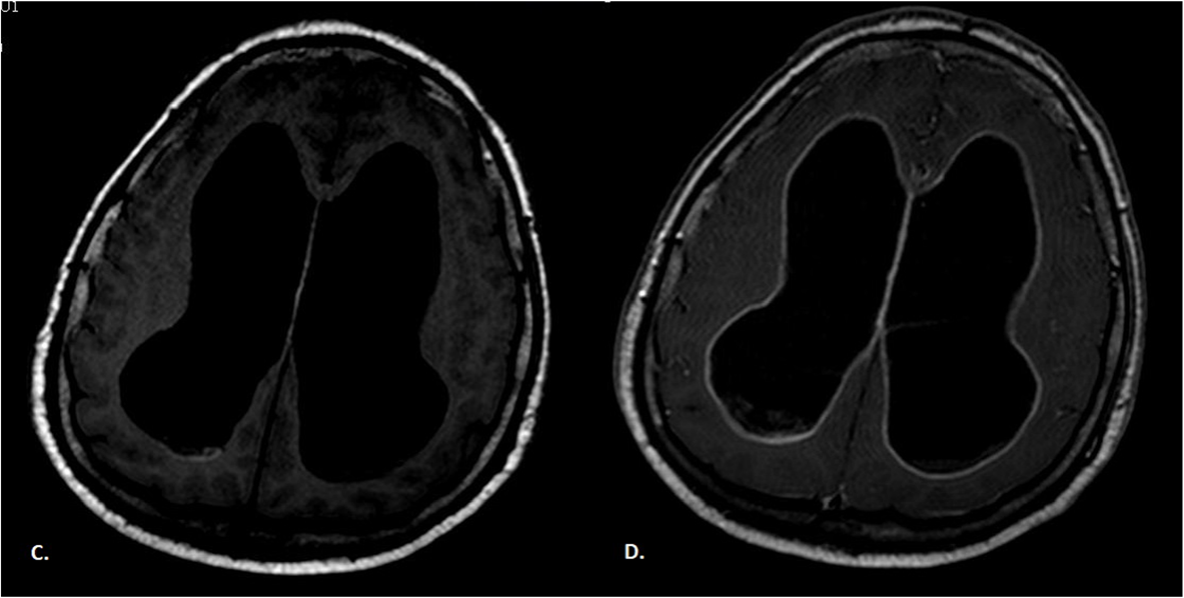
Brain MRI T1-weighted imaging without (c) and with (d) gadolinium contrast enhancement. Hydrocephalus as a consequence of disease progression. Hyper-intensity on T1 was due to the paramagnetic effect of melanin, which had stable organic free radicals inside it, resulting in shortened T1 relaxation times in typical melanotic melanoma
